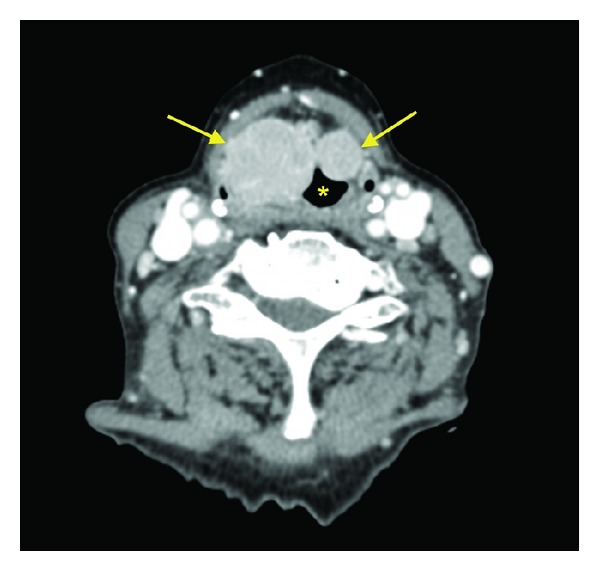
Axial contrast enhanced CT scan at the level of true vocal cords showing bilateral solid paraglottic masses (arrows) narrowing the laryngeal lumen (asterisk).
